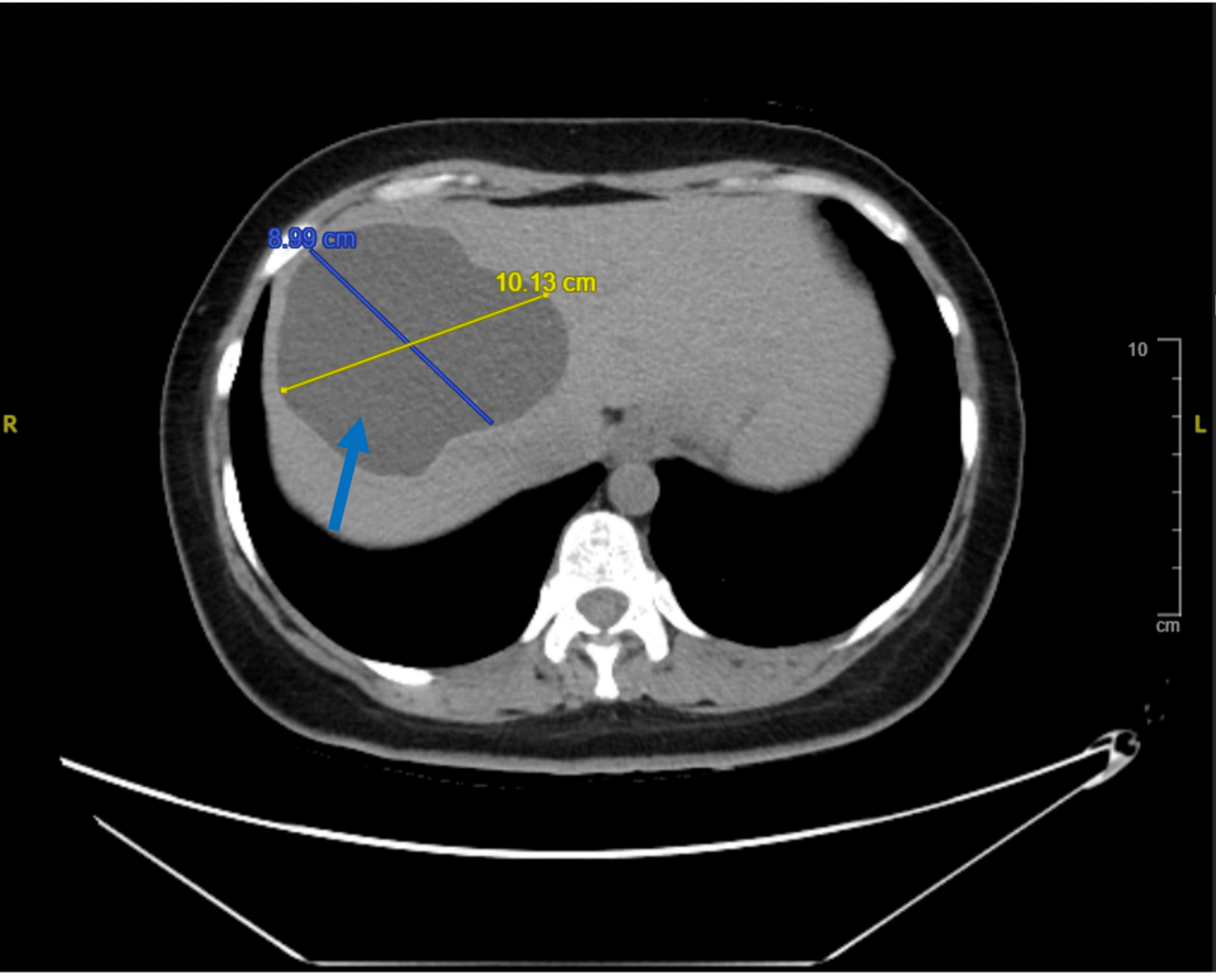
Axial View of a Non-enhanced Abdominal Computed Tomography Scan. Axial view of a non-enhanced abdominal computed tomography scan showing hepatomegaly with a large right hepatic lobe thick-walled fluid-filled hypodense cystic lesion (blue arrow).
